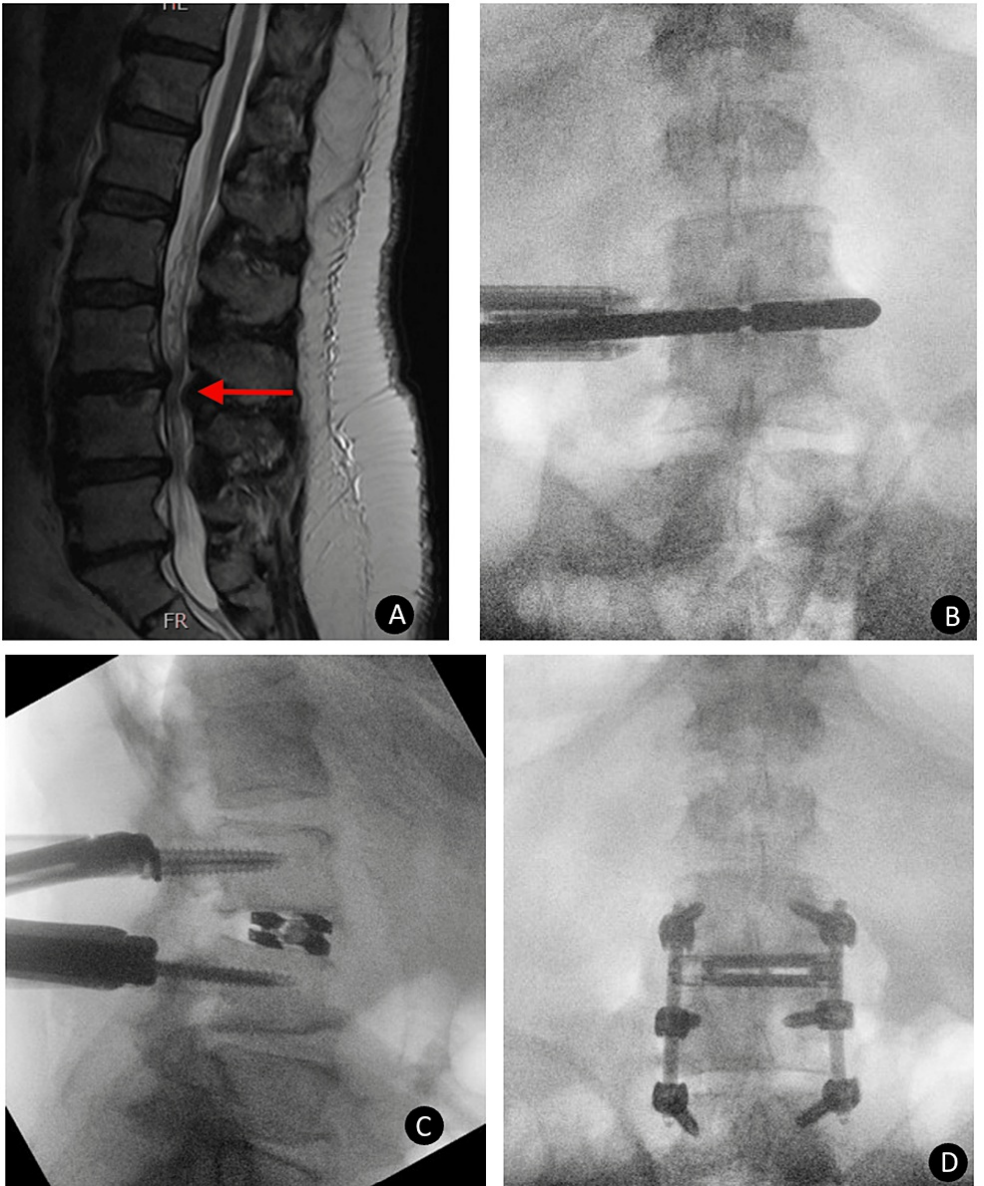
Case 3 postoperative radiographic evaluation of dual expandable spacer and screw placement A 69-year-old male presented with lumbar radiculopathy and low back pain. Preoperative sagittal MRI (A) showing multilevel degenerative changes of the lumbar spine with multilevel central spinal stenosis, severe at L3-4 (indicated by the red arrow). Intraoperative AP (B, C) x-ray image showing steps in the placement of posterior fusion hardware extending from L3-L5 with an interbody spacer at L3-L4. Postoperative AP (D) x-ray image showing posterior fusion hardware extending from L3-L5 with an interbody spacer at L3-4 with maintained disc space height and no subsidence.
